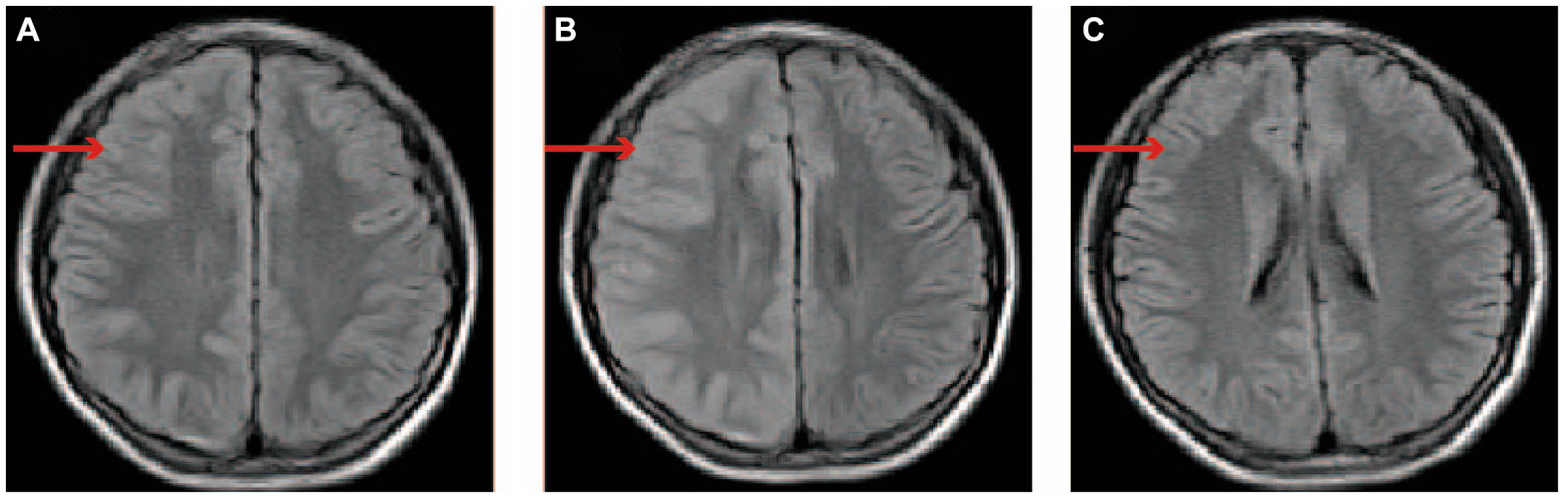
The changes of brain MRI images. (A) On day 1 following the onset of symptoms, the brain’s MRI displayed unremarkable findings. (B) On day 12 after symptom onset, the brain enhancement MRI is characterized by thickening of the cerebral cortex in the right cerebral hemisphere, the widening of the gyri, and the disappearance or shallowness of the sulci. (C) On day 45 after symptom onset, the MRI of the brain returned to its normal state. The red arrow indicates the right cerebral cortex. MRI: magnetic resonance imaging.
